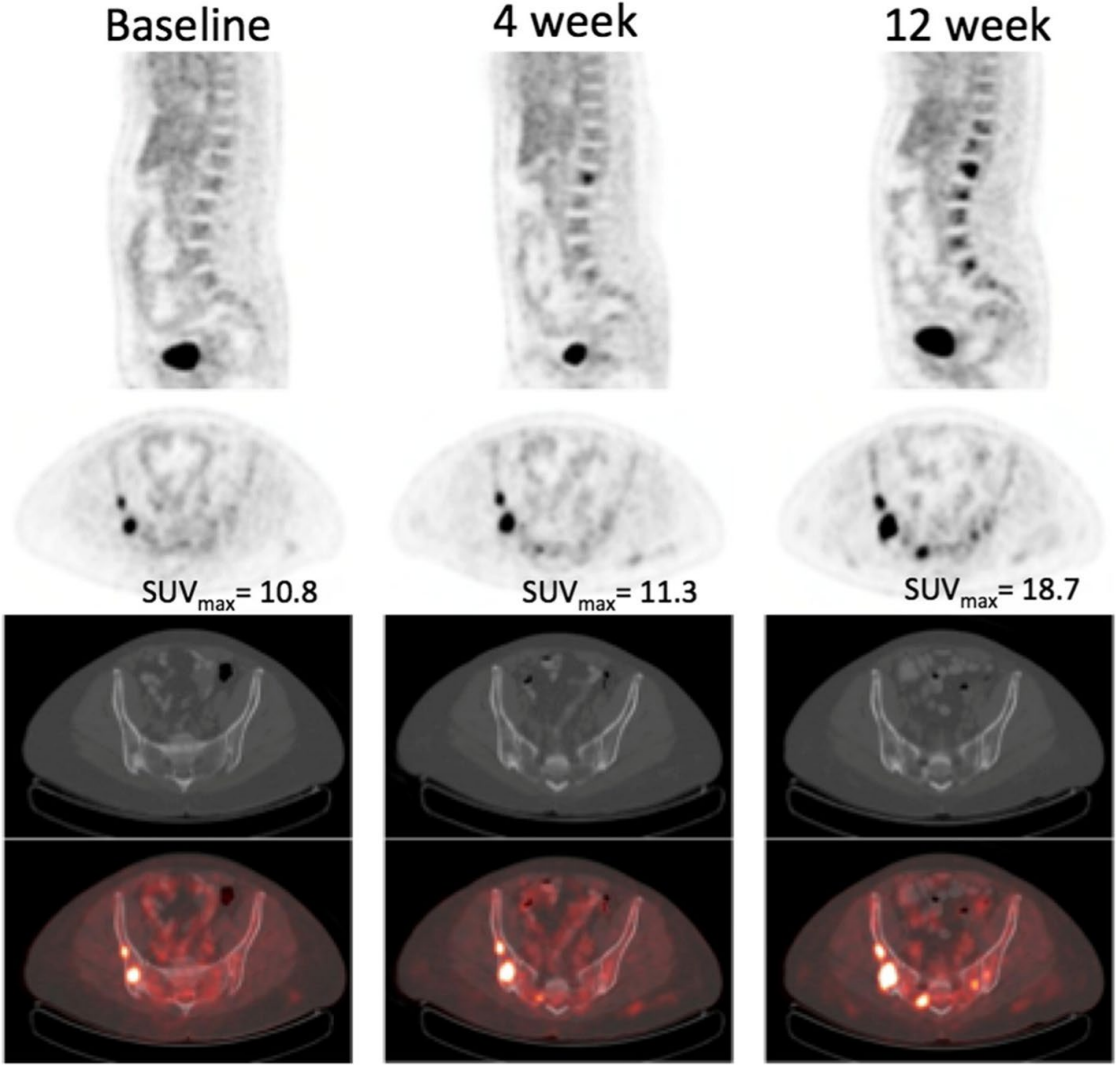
This patient with ER-positive breast cancer started a new endocrine therapy. Her FDG-avid osseous lesions did not demonstrate a decrease in FDG uptake to indicate a metabolic response at 4 weeks and 12 weeks. The patient progressed relatively quickly, in just over 100 days
